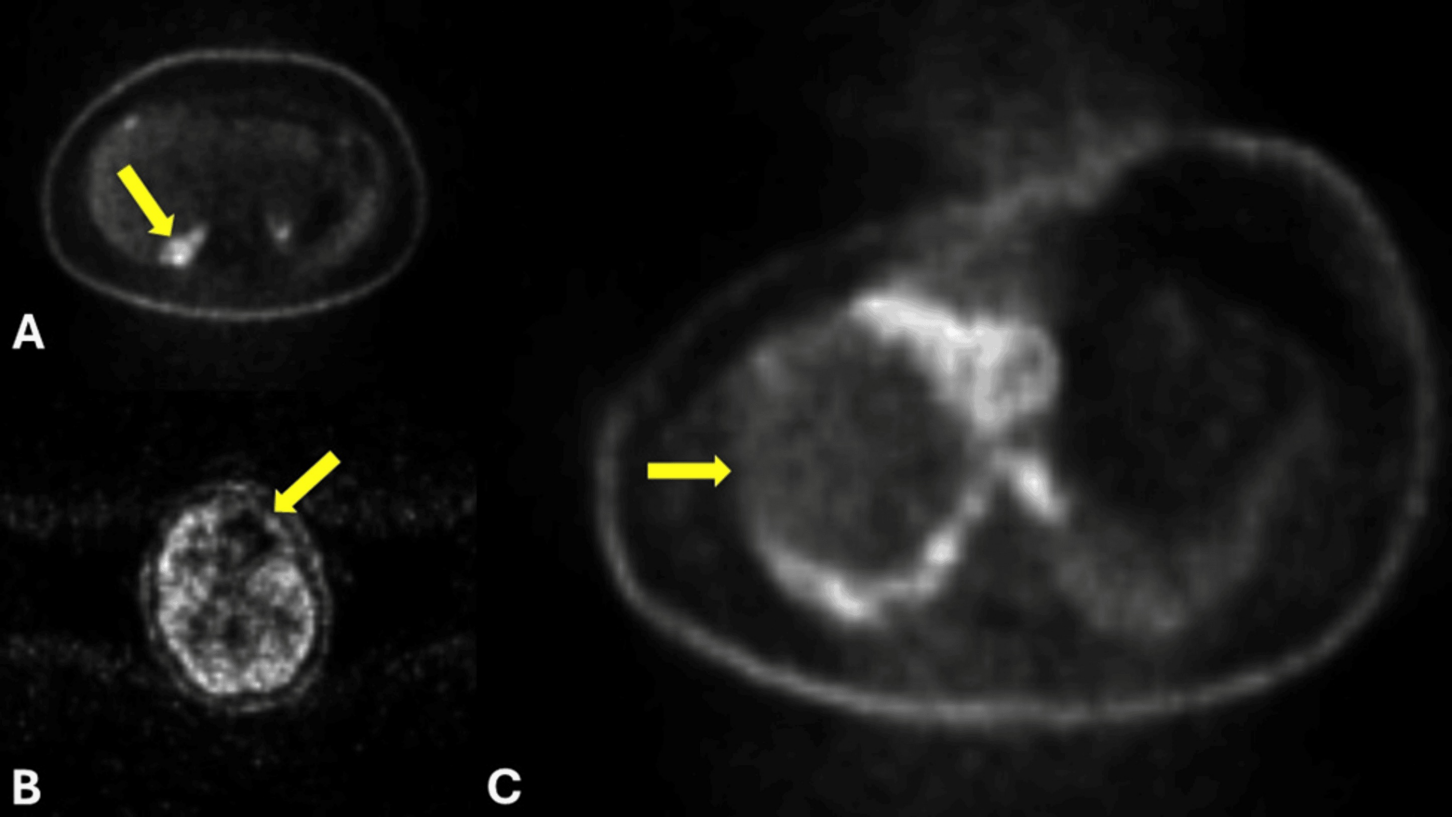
Positron emission tomography with metastasis. Cross-sectional positron emission tomography (PET) imaging with IV contrast showing (A) an axial view demonstrating hypermetabolic activity in the right hepatic lobe adjacent to the gallbladder (yellow arrow) (SUVmax 7.4), (B) an axial view demonstrating a large abnormal photopenic area in the left frontal lobe (yellow arrow), and (C) an axial view demonstrating hypermetabolic activity in the right middle lobe of the lung (yellow arrow), measuring 7.5×6.9×11 cm, with central necrosis and displacement of the mediastinum to the left (SUVmax 18). SUV: standardized uptake value
